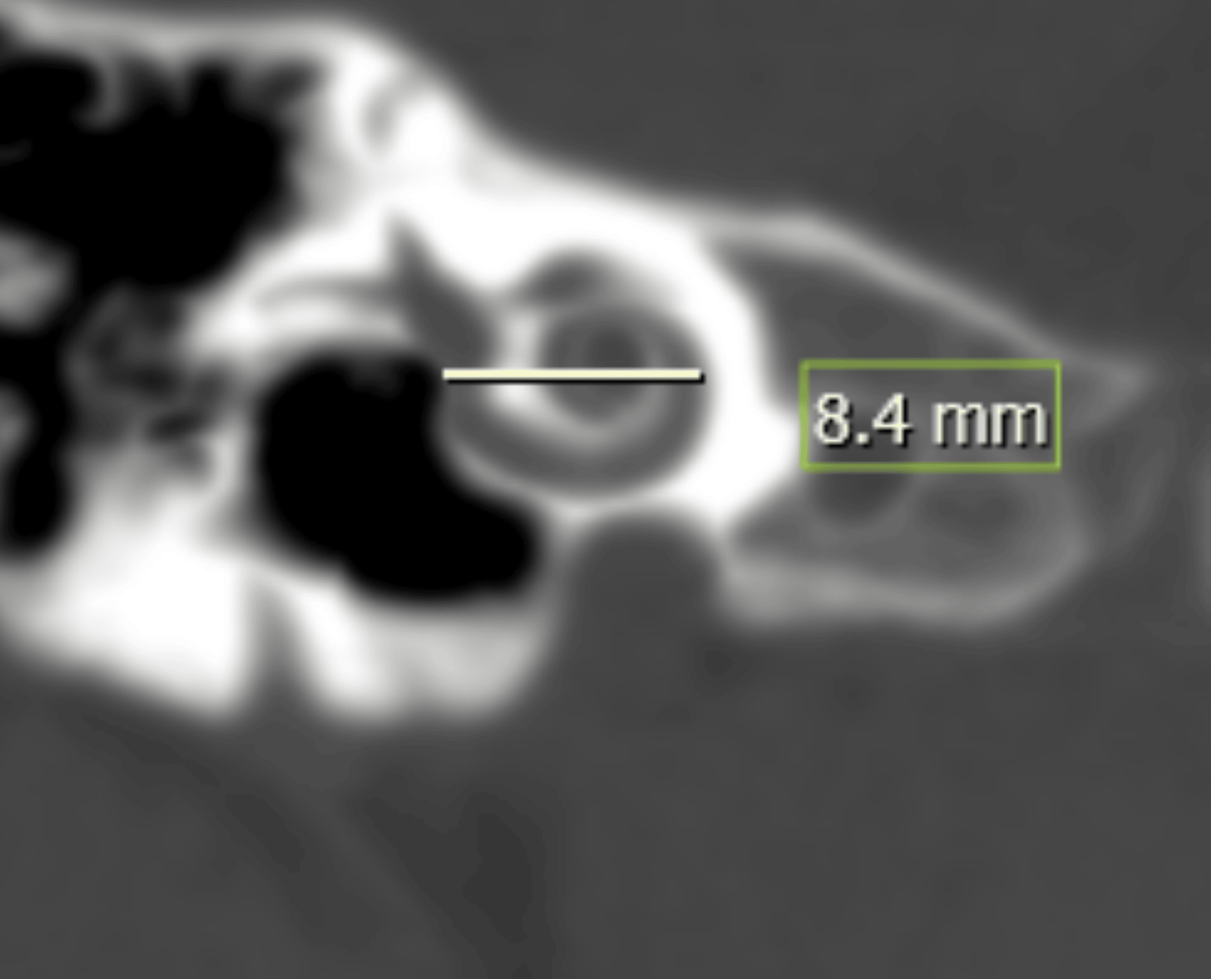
Cochlear duct length (CDL) measurement. A-value = distance from the round window through the modiolus to the opposite lateral wall on mid-modiolar CT (white line).
